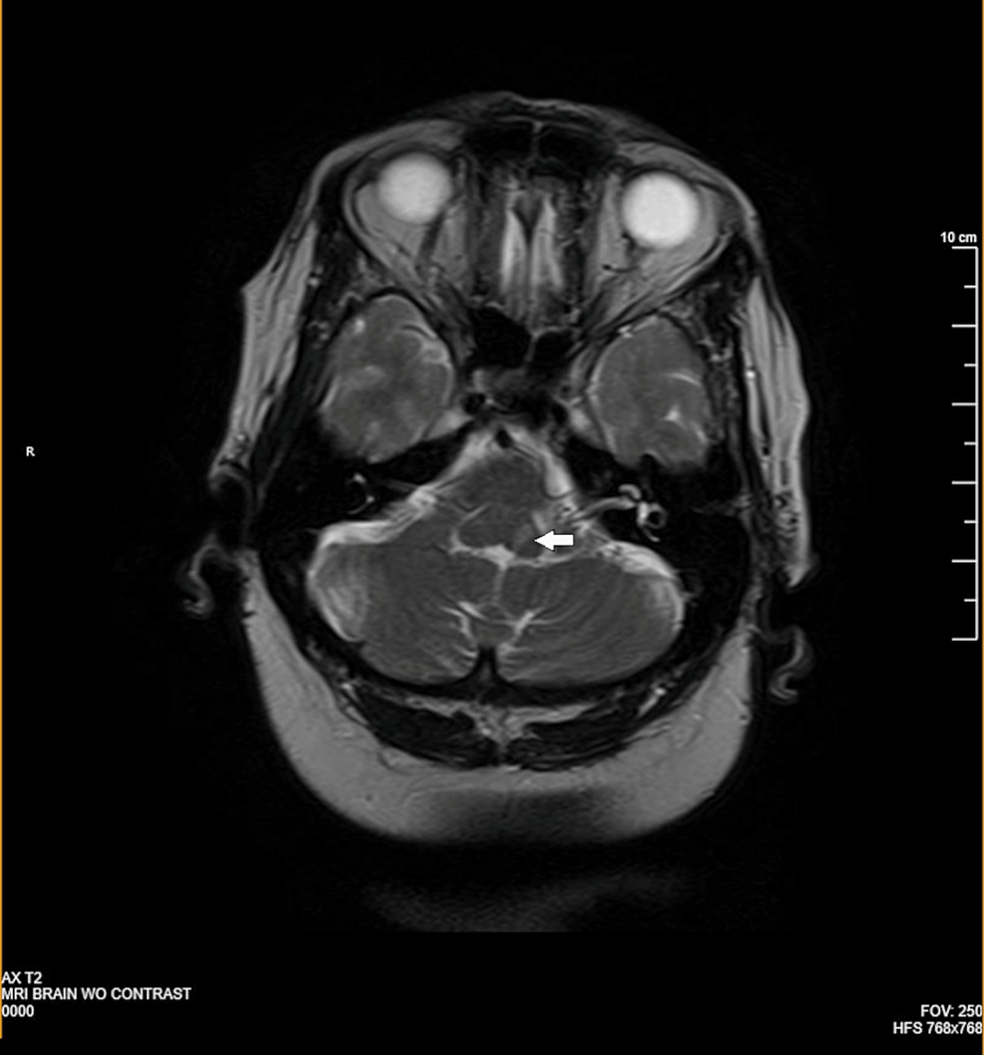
MRI brain showing small left dorsal brainstem infarct at the pontomedullary junction (white arrow)
